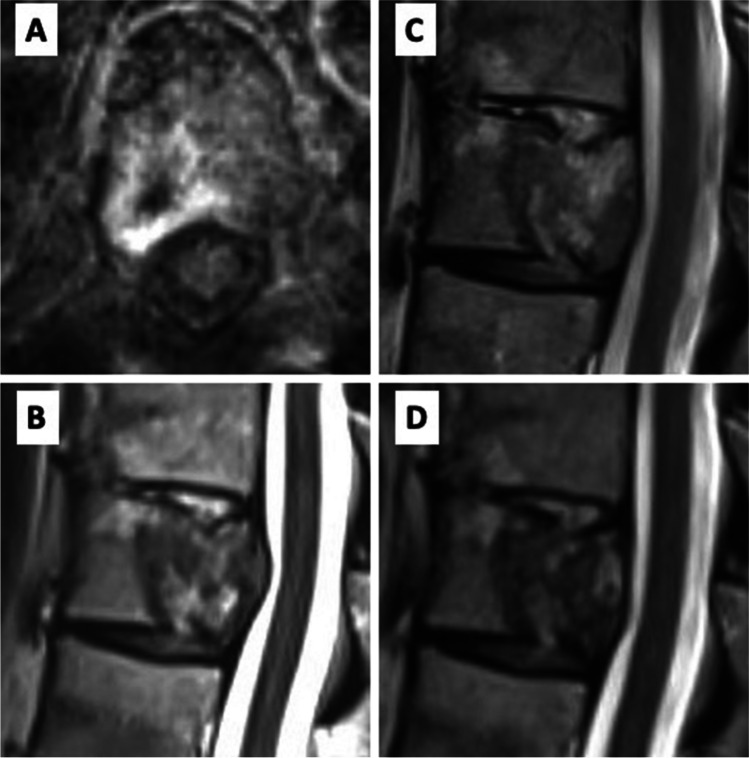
A) axial MRI T1+Gd 72h after surgery, B) sagittal MRI T2 after 3 months C) sagittal MRI T2 after 6 months D) sagittal MRI T2 after 10 months
